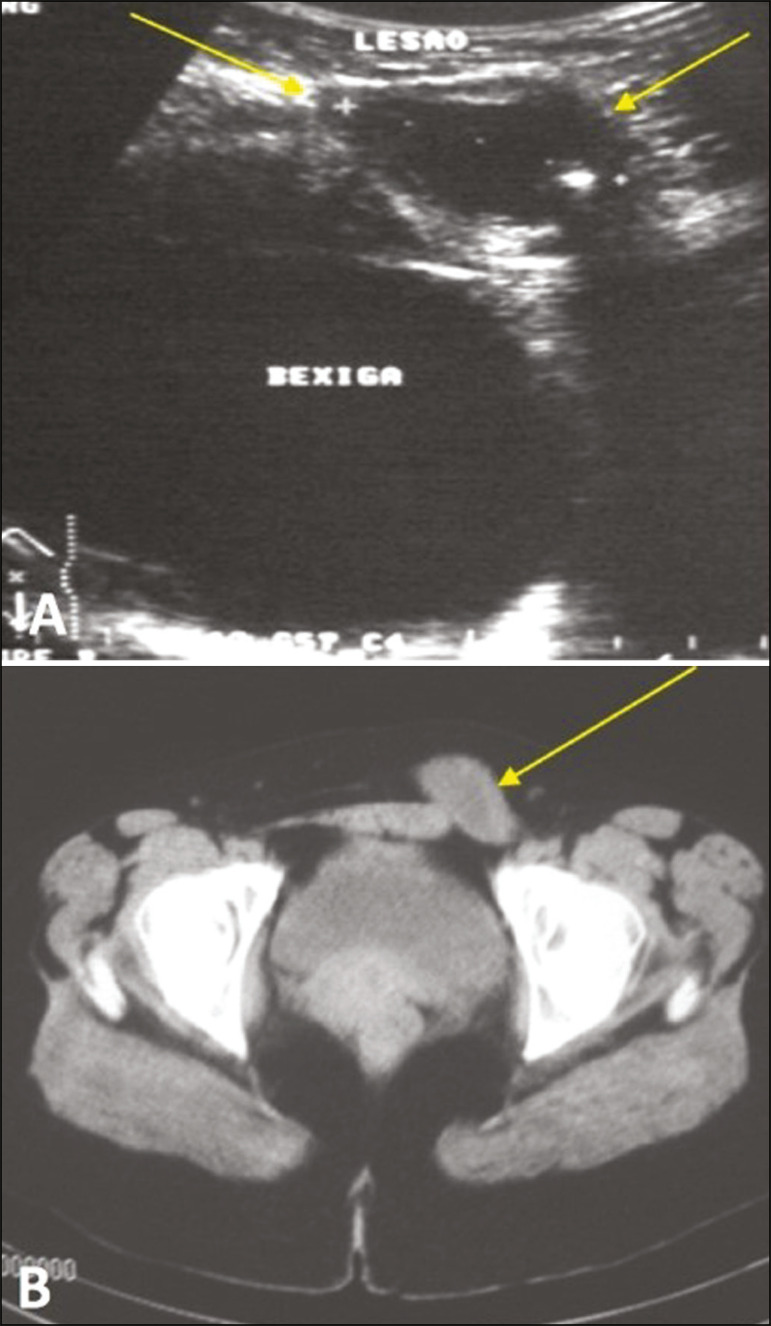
A 28 year-old female with a painful mass, which proved to be an ovarian cyst within an inguinal hernia, in the left inguinal region. A: Ultrasound showing an ovarian cyst in the inguinal canal. B: Axial CT in the portal phase showing that cyst (arrow). Image courtesy of Dr. Lutero Marques de Oliveira.
